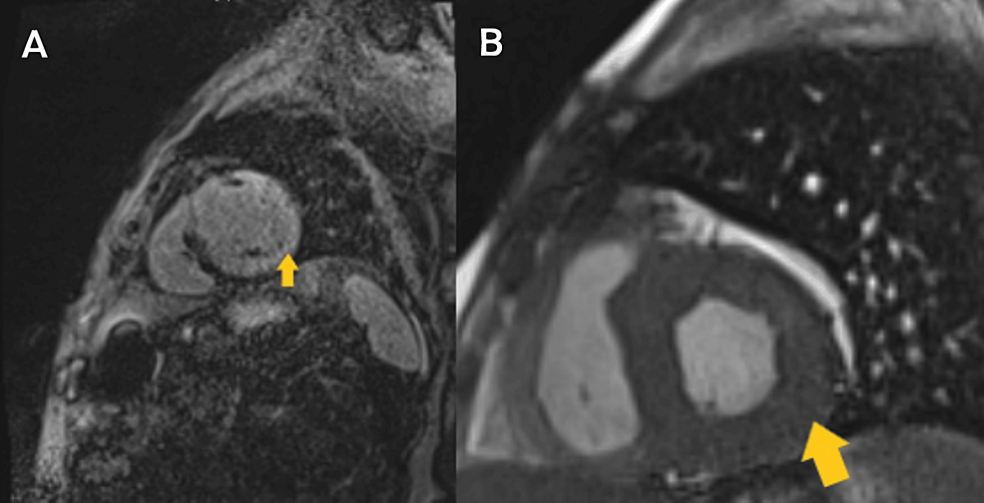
Cardiac magnetic resonance imaging - sagittal view (a) diffuse subendocardial late gadolinium enhancement and (b) basal short-axis steady-state free precession (SSFP) concentric left ventricular hypertrophy.
